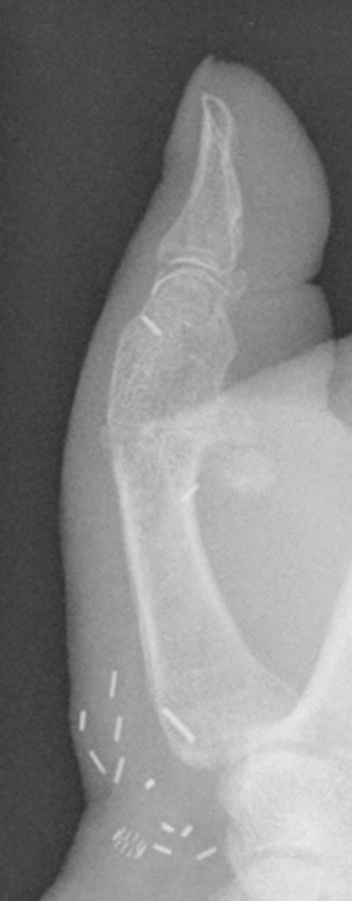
X-ray of the right thumb 7 months after the end of the antibiotic treatment
